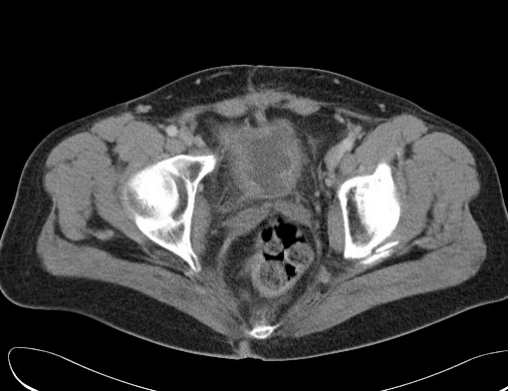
A computed tomography scan showing a thickening of the entire bladder wall with no predominant location, such as the bladder dome.
